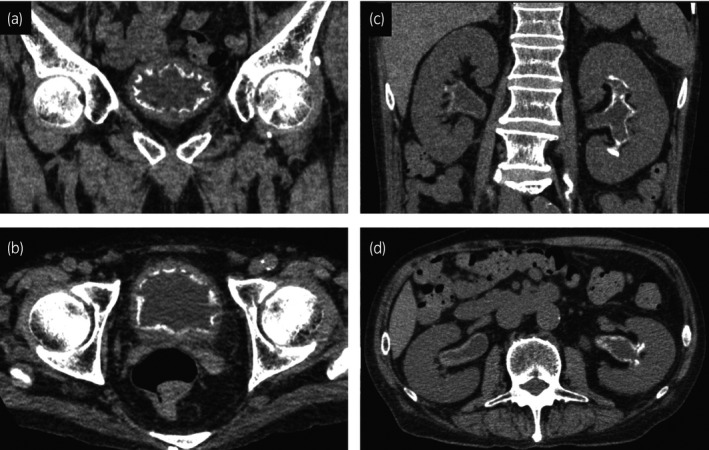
Coronal and axial CT images of bladder (a,b) and kidney (c,d). A thin layer of calcification along the bladder and pelvic wall was observed.
